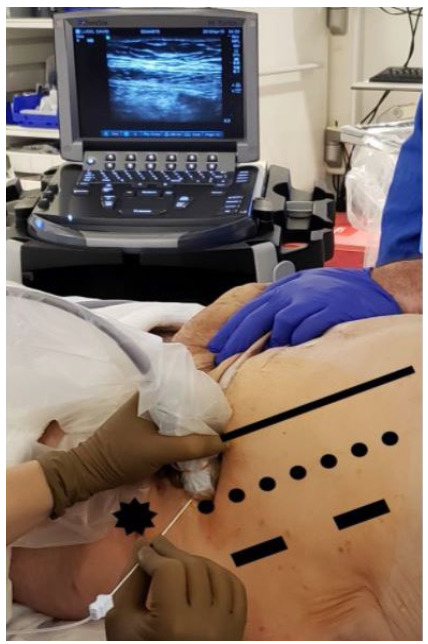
Ultrasound-guided BTA injection demonstrating a view of the left lateral abdominal wall demonstrating the landmarks for the injection sites. Anterior Superior Iliac Crest (Black Star), Semilunaris line (Black Line), Anterior Axillary line (dotted line) Mid Axillary line (Black Rectangles).
